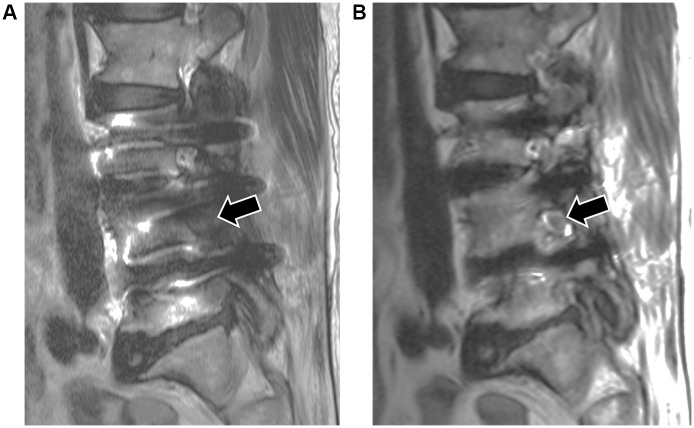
Visibility score of neural foramen and diagnostic confidence of neural foraminal stenosis in sagittal images. These two sagittal images are obtained from a 75 year old female who underwent inter-body fusion at L3-4 and L4-5 and posterior instrumentation at L3-L5 level due to spinal stenosis. The time interval between surgery and the spine MRI was 2 years and 6 months. (a) Sagittal Fast spin echo T2 -weighted image shows grade 0 (observer 1) and 0 (observer 2) of visibility of neural foramen at the left L4-5 (arrow). (b) Sagittal FSE-SEMAC T2- weighted image shows grade 3 (observer 1) and 2 (observer 2) of visibility of neural foramen at the same level (arrow). After application of FSE-SEMAC sequence, diagnostic confidence level of neural foraminal stenosis was changed from grade 3 (inconclusive) to grade 1 (definitely absent) in both reviewers.
